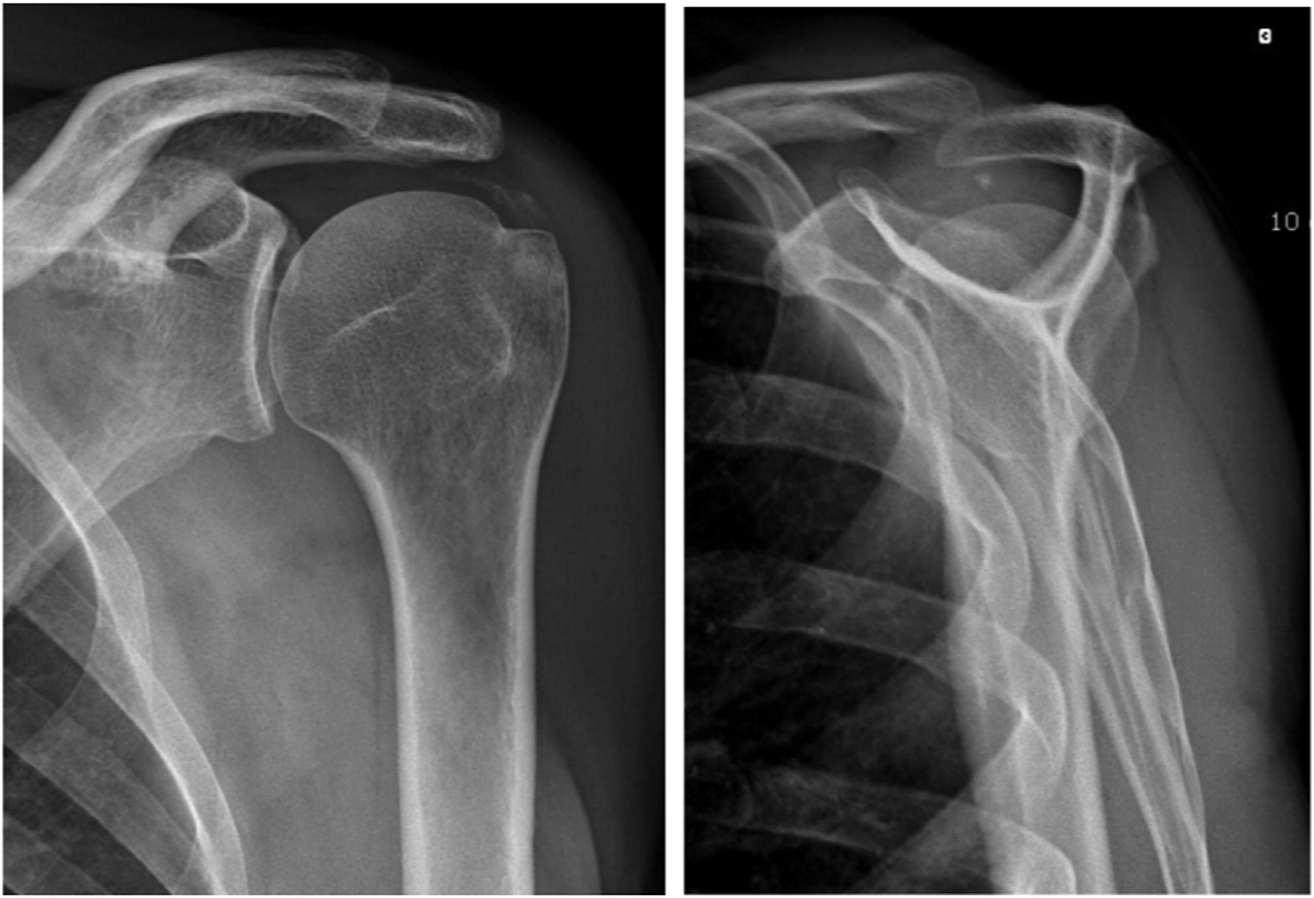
Anterior-posterior X-ray on the left and scapular Y profile of the patient on the right with an aspect of heterogeneous calcification of the supra spinatus tendon.
